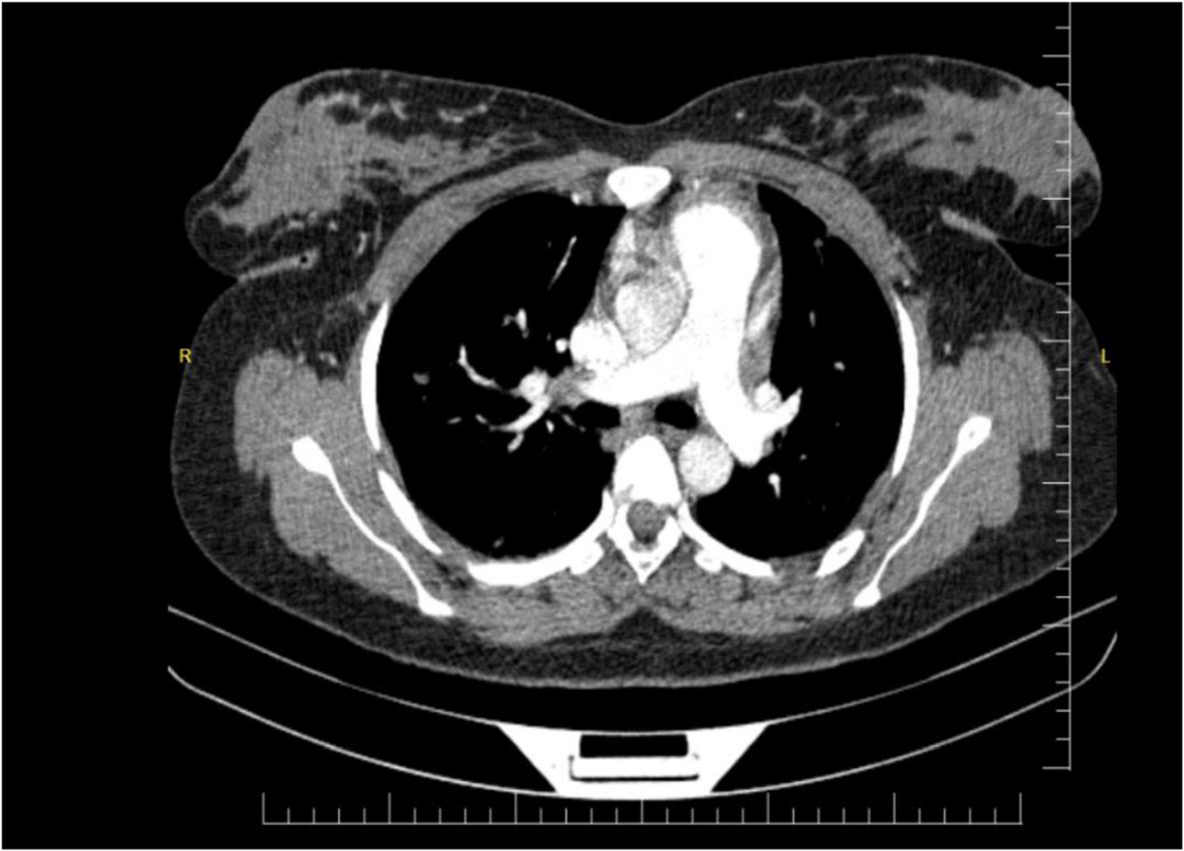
Computed tomography pulmonary angiography revealed normal pulmonary vessels with no thrombus or emboli
